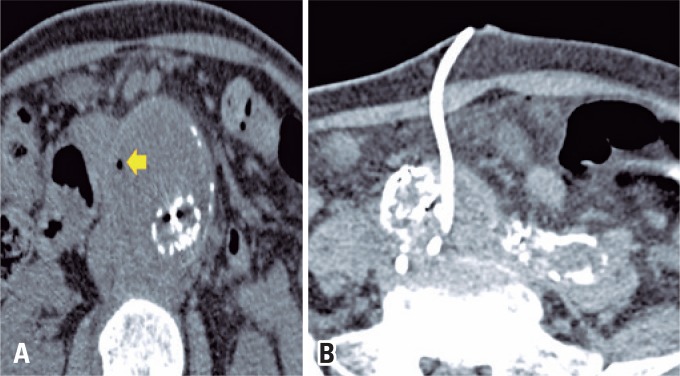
Drainage of peri-prosthetic infected fluid collection. (A) Non-contrast full abdominal CT scan: aneurysm sac with peri-prosthetic fluid collection with gas (arrow); (B) Post-drainage CT: proper placement of the drain, and reduced collection
